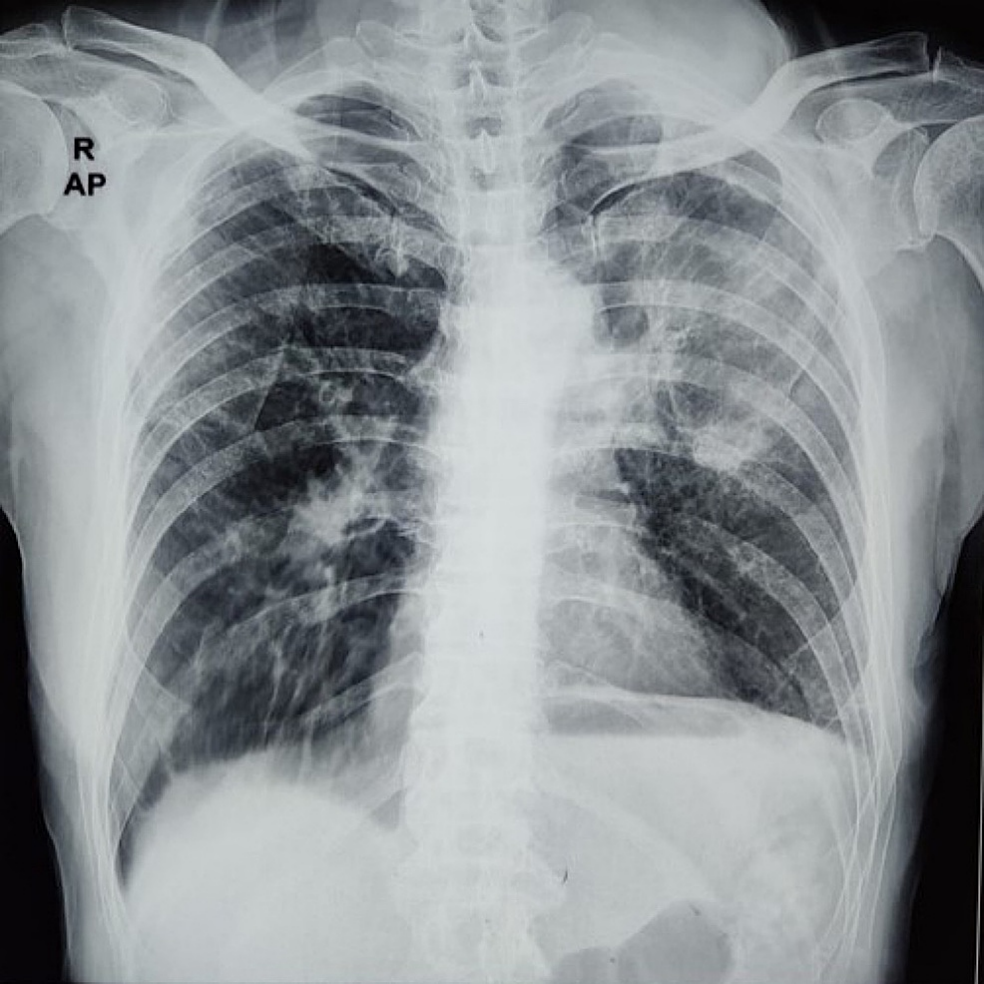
Chest X-ray postero-anterior view suggestive of a large opacity on the left side chest.
